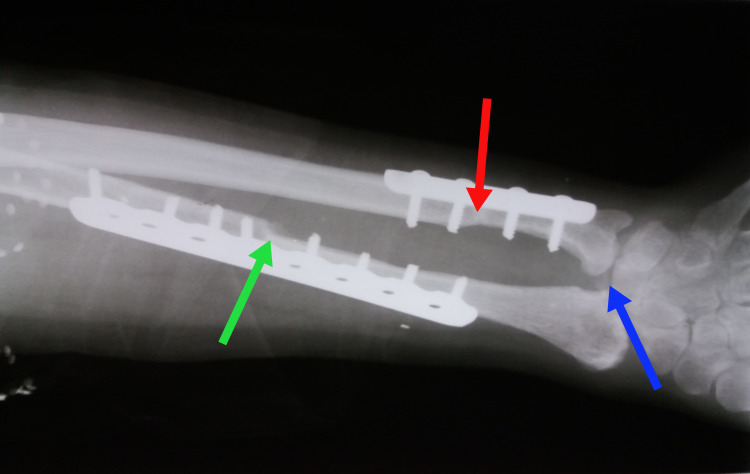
X-ray at the one-year follow-up showing good union of the graft with residual radius (green arrow), complete union of shortened ulna (red arrow), and the moderate distraction of radioulnar joint (blue arrow).
